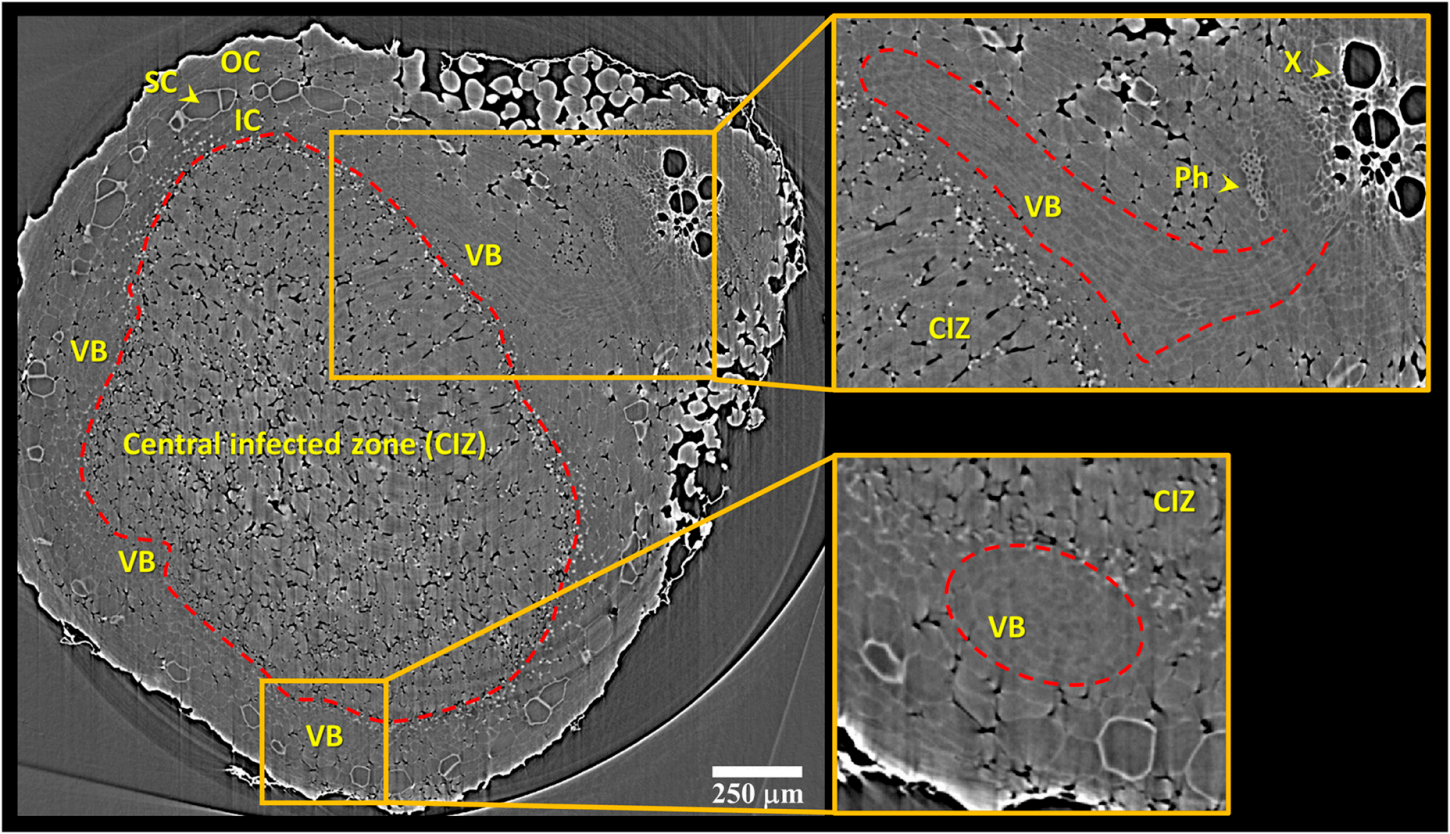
Synchrotron x-ray micro-tomogram of a fresh root nodule of a 4-week-old soybean plant (genotype PI209332). The water-filled cells from various tissues within the root and nodule appear gray, while air-filled intercellular spaces, particularly noticeable in the large embolized xylem vessels (X) within the root, appear black, due to their lower x-ray attenuation. The central infected zone (CIZ), outlined in red, is readily identified by the tightly packed non-infected parenchyma cells of the boundary layer that surrounds it. Magnified portions of the tomographic nodule section are shown in inset boxes, with the VB’s outlines highlighted in red. The scleroid layer (SC), separating the inner (IC) and outer (OC) cortices, is labeled.
